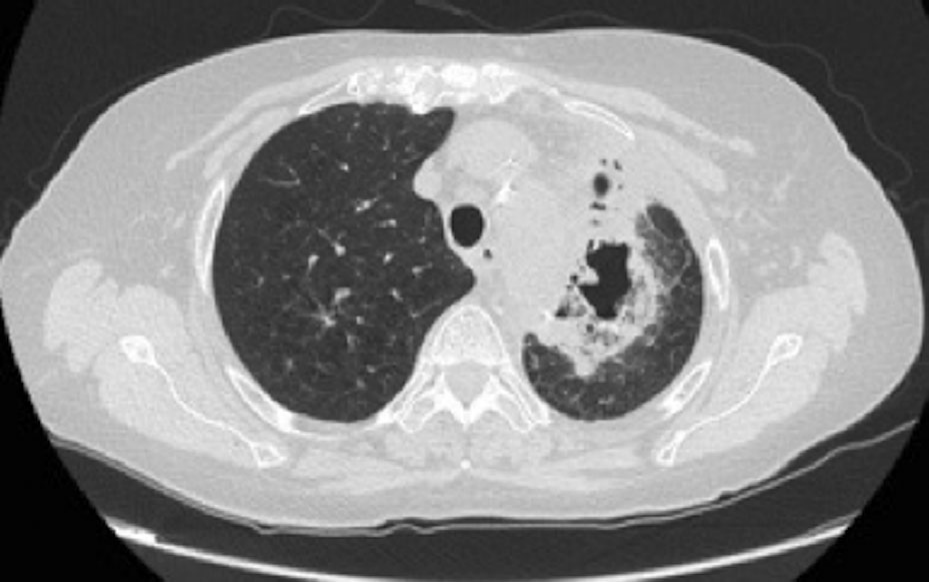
Cavitary left lung lesion detected on post‐operative chest computed tomography.
